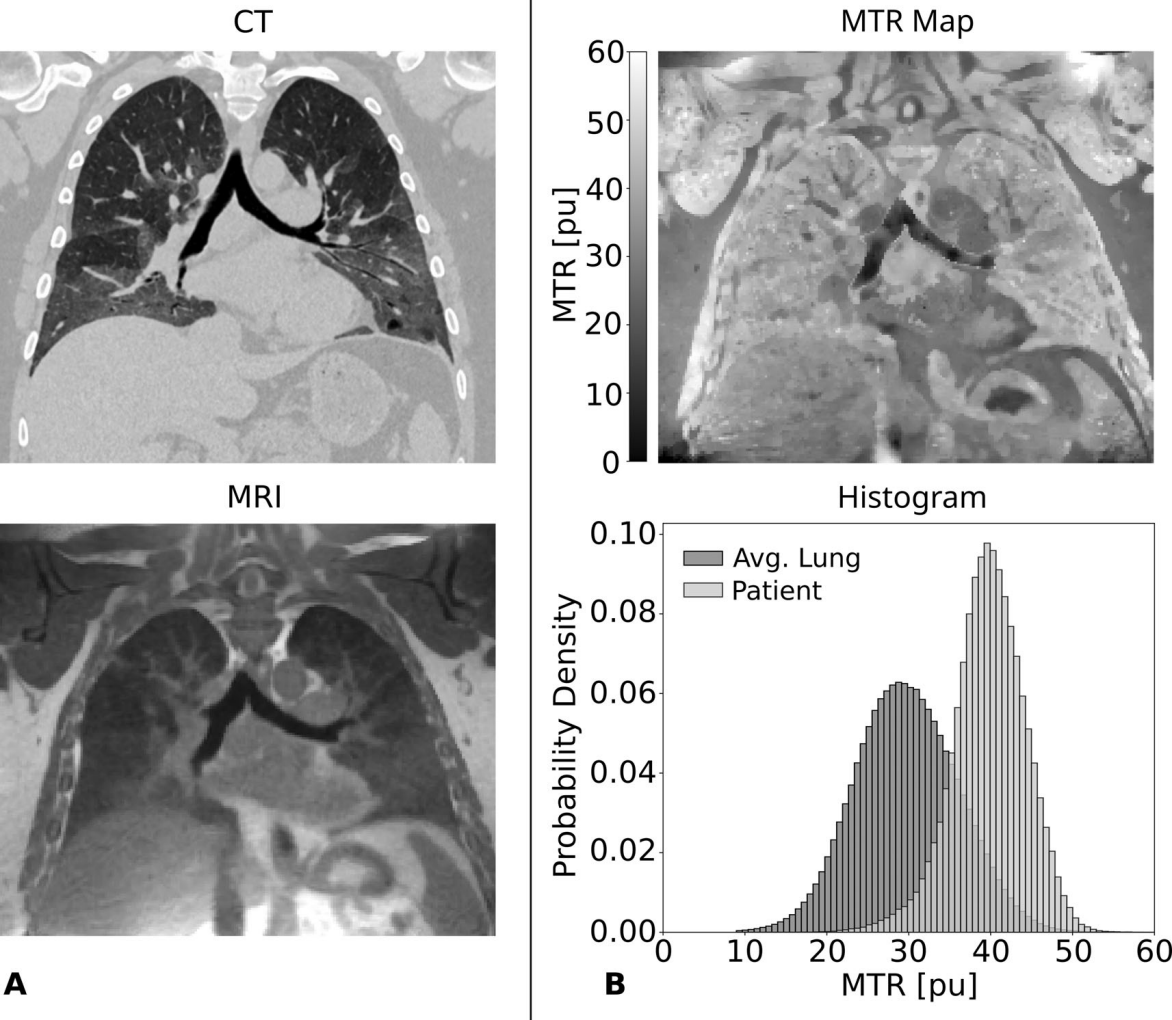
Patient with chronic thromboembolic pulmonary hypertension (CTEPH): (A) morphological images: CT and MRI, and (B) MTR maps and whole lung MTR histogram for the patient in light gray and collective data of healthy volunteers, representing an average healthy lung, in dark gray.
